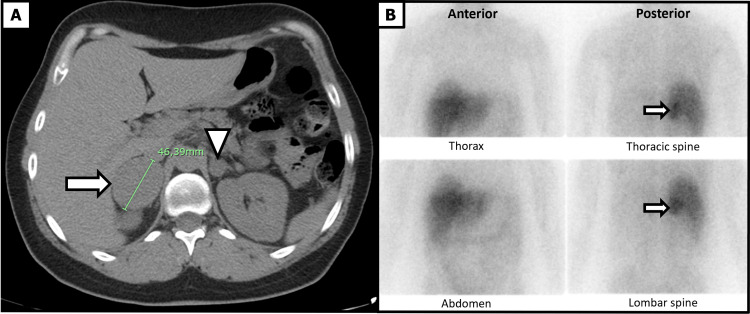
Bilateral adrenal lesions on CT and MIBG scintigraphy A) Axial CT scan showing a right adrenal lesion (horizontal white arrow) and a partially visualized left adrenal lesion (arrowhead). B) Iodine-131-MIBG scintigraphy demonstrating intense focal uptake in the right adrenal lesion (horizontal white arrow). On the left side, only a very faint, low-grade activity was observed in the expected adrenal topography, with intensity markedly lower than hepatic background, and interpreted as likely physiological adrenal uptake rather than evidence of a focal lesion. MIBG - metaiodobenzylguanidine
